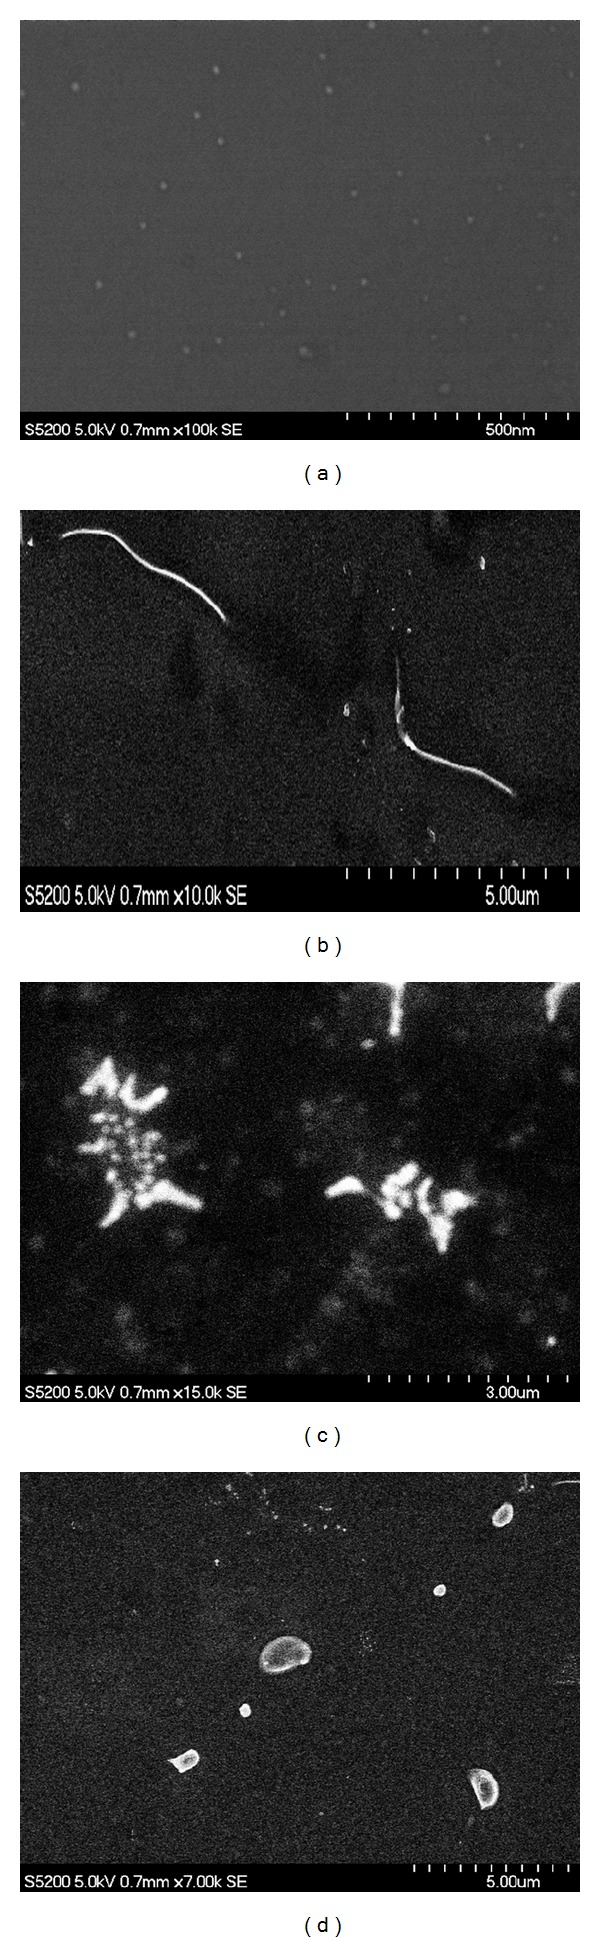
SEM images of CT-DNA at different concentrations of AuNPs in the presence of [NaCl] = 0.015 M. (a) [AuNPs] = 1 × 10^−6^ M; (b) [DNA] = 2 × 10^−6^ M; (c) [DNA] = 2.5 × 10^−6^, [AuNPs] = 2.5 × 10^−7^ M; (d) [DNA] = 2 × 10^−6^ M, [AuNPs] = 1 × 10^−6^ M.
